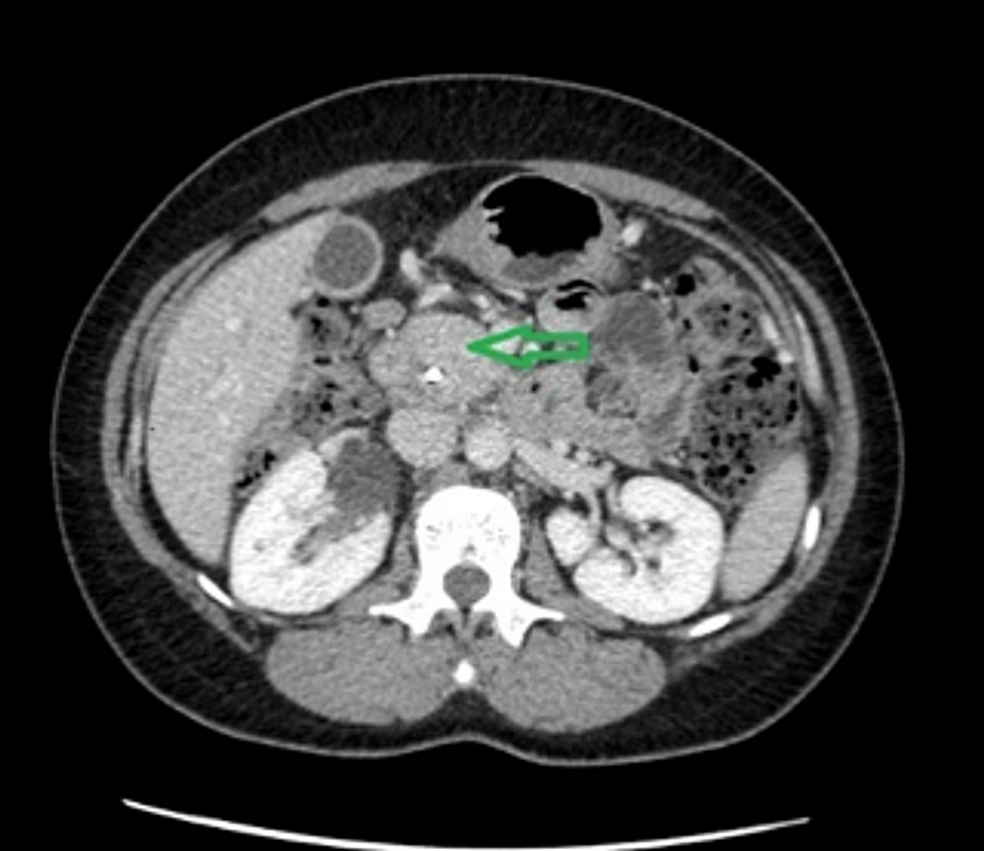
On day 21 follow-up post-steroid treatment, CT angiogram of the chest and abdomen revealed a pancreatic head measuring approximately 3.2 × 1.6 cm, an improvement from 4.3 × 3 cm. The pancreatic body (green arrow) at the current examination measured approximately 1.8 cm in its thickness improving from 2.8 cm. CT: computed tomography
